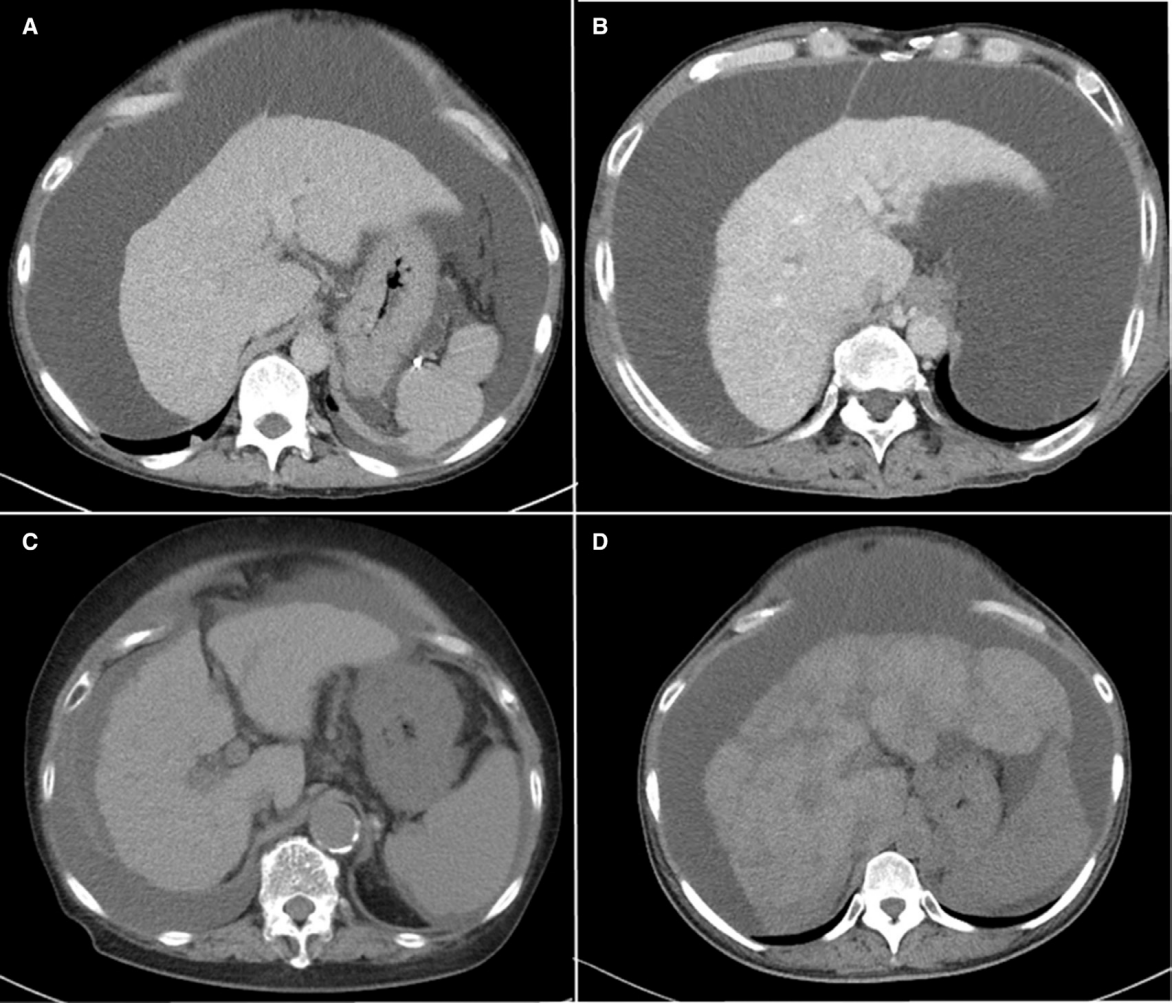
Abdominal computed tomography scan images before TIPS creation. (A) Case 1, (B) case 2, (C) case 3 and (D) case 4. TIPS, transjugular intrahepatic portosystemic shunt.
